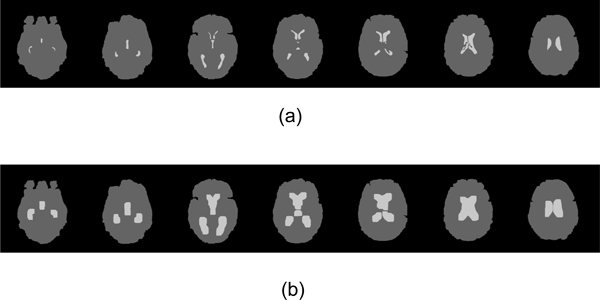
MRI template set after dilation. (a) The bright parts are the original MRI template including two lateral ventricles and third ventricles. The gray parts are the brain parts. (b) In order to accommodate the variations of ventricle positions in pathological cases, the template slices are enlarged by morphological dilation operation.
